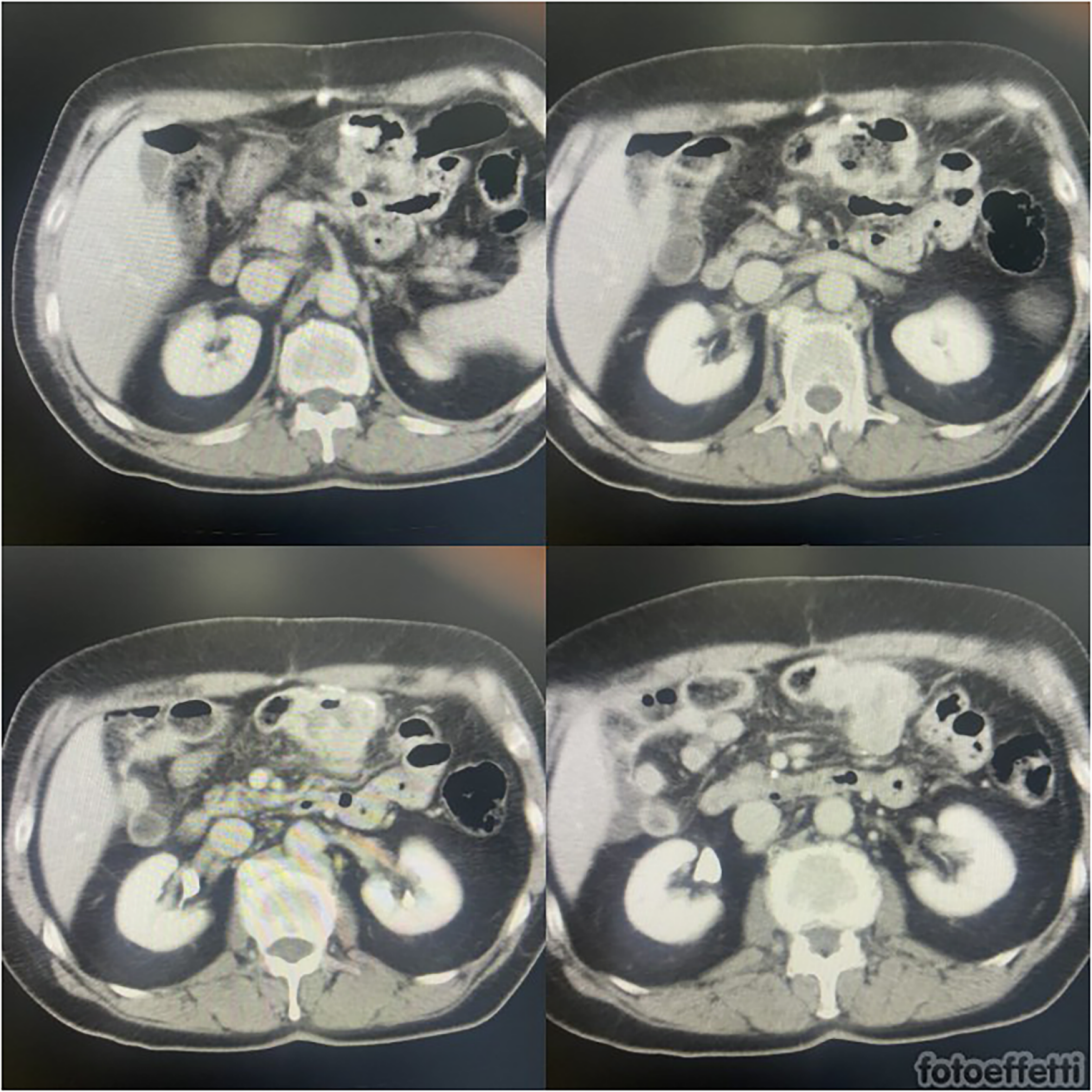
CT assessment of colon metastasis detected during follow-up.
